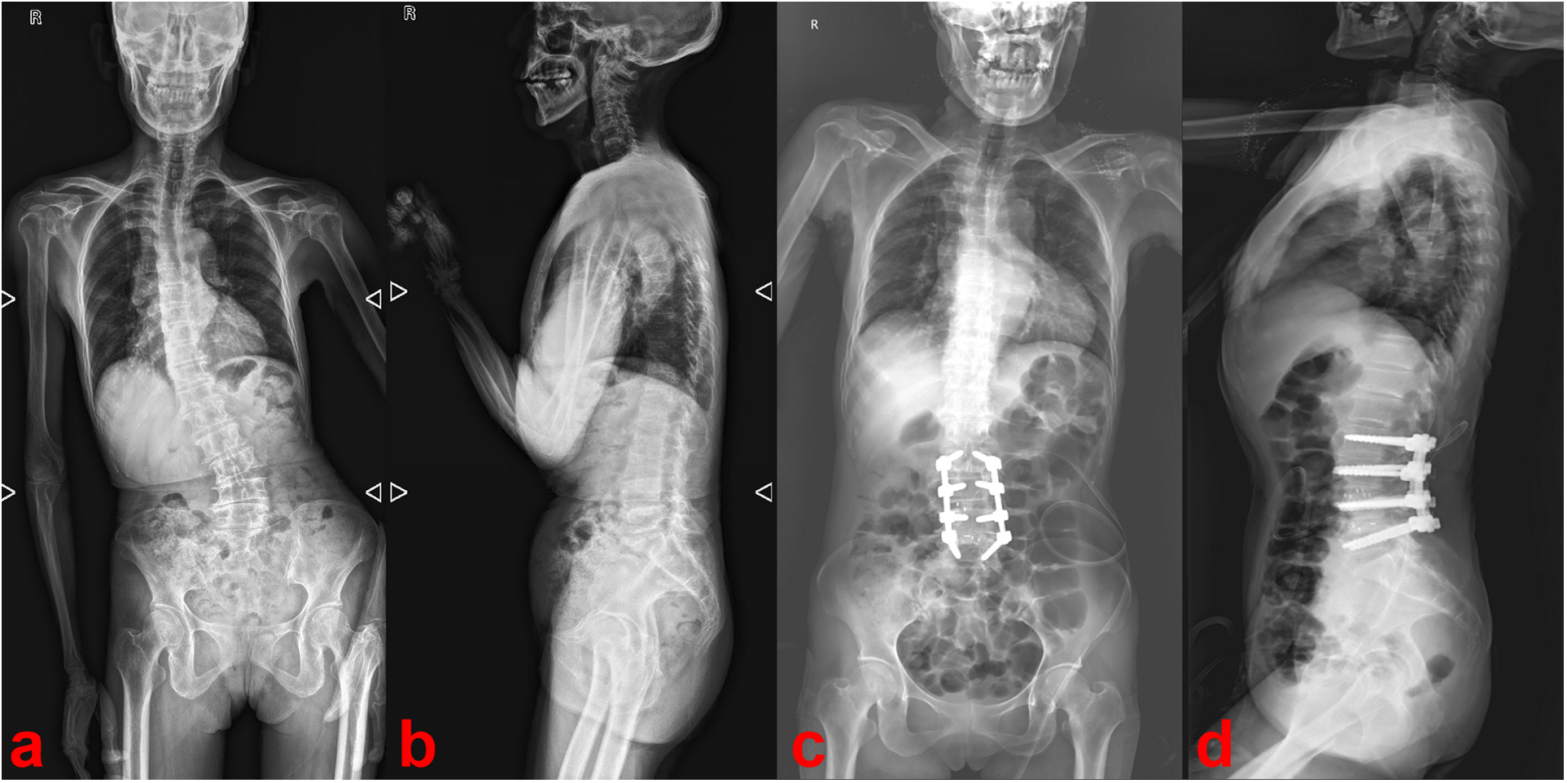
Preoperative (a,b) and postoperative (c,d) radiographs of the full-length spine.
